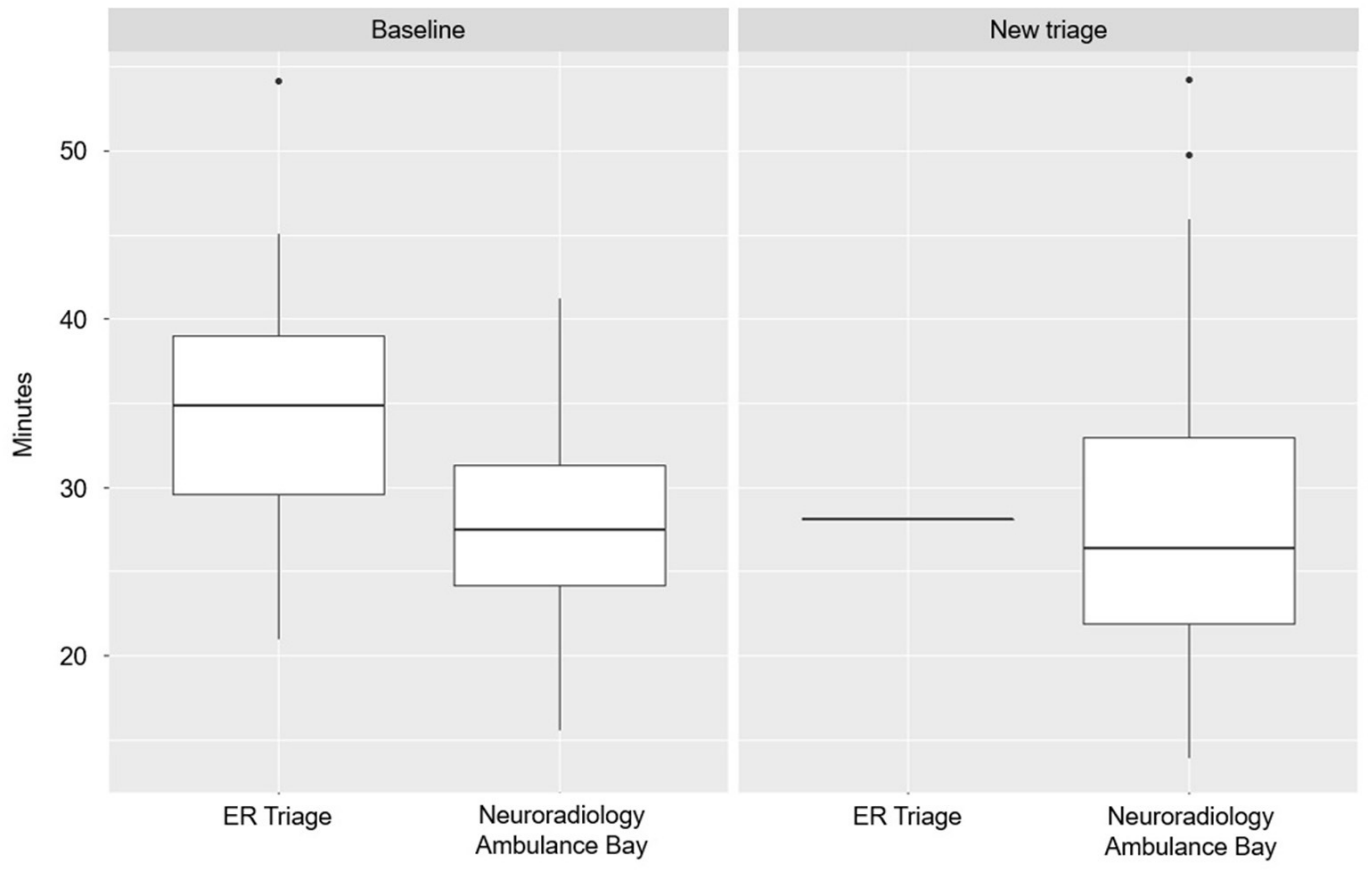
Time from hospital arrival to leaving the CT room comparing entry points (ER emergency room or neuroradiology ambulance bay).
